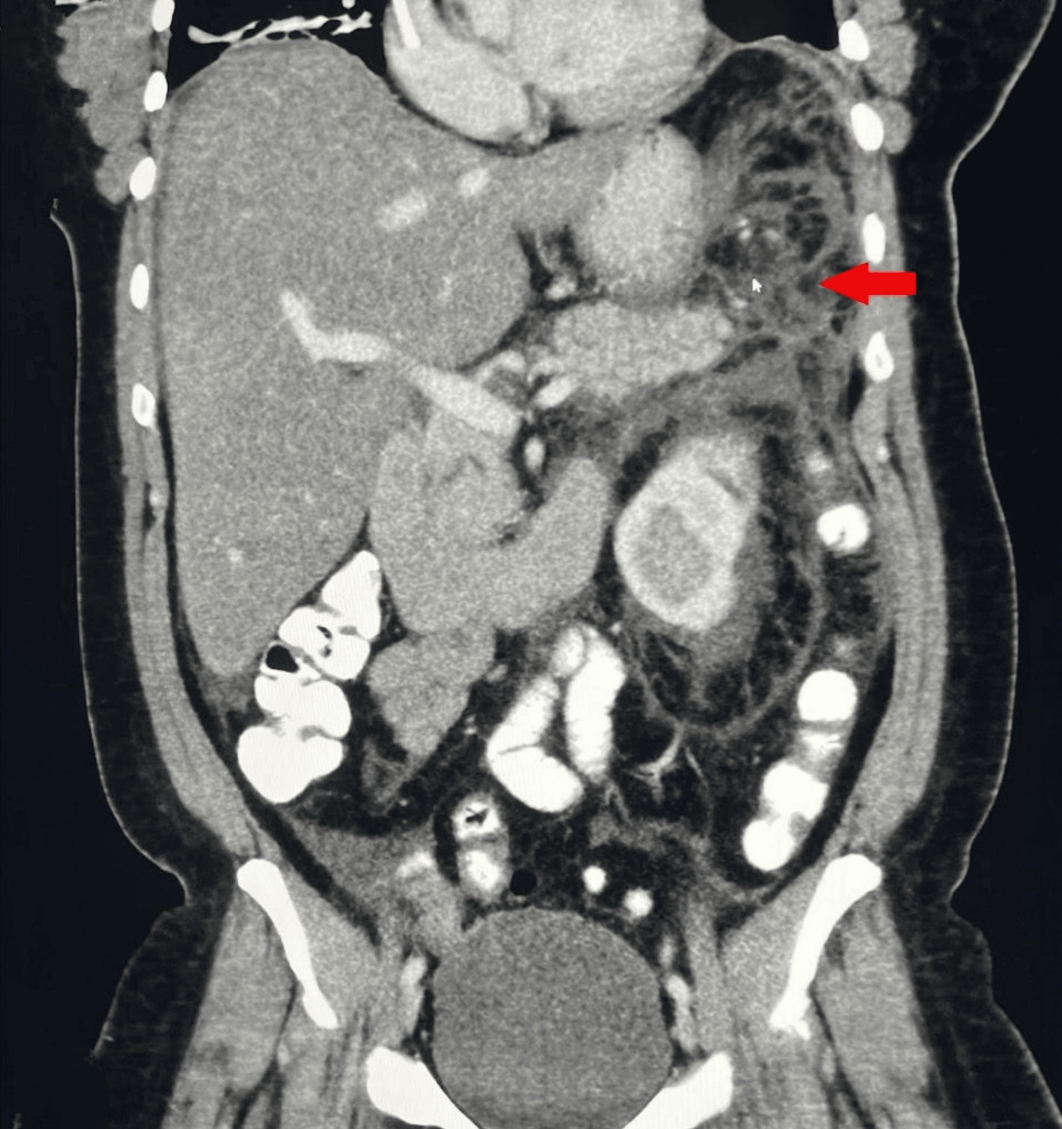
The coronal view of the CT scan reveals significant pancreatic inflammation consistent with acute pancreatitis (indicated by the red arrow) CT: Computed tomography
